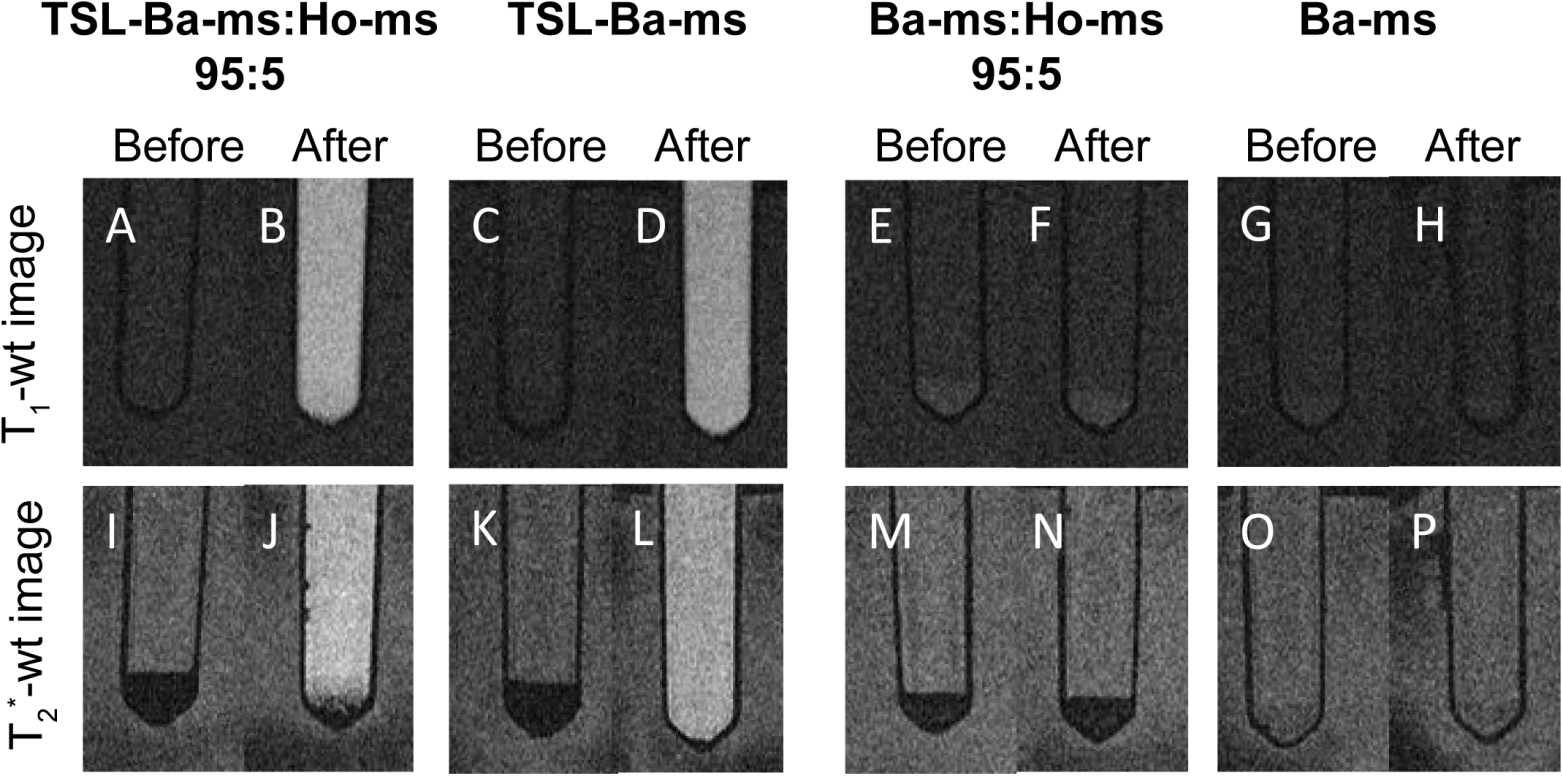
T_1_ and T_2_*-weighted MR images of TSL-Ba-ms or Ba-ms mixed with or without Ho-ms (ratio 95:5) before mild hyperthermia. T_1_ and T_2_*-weighted MR images of TSL-Ba-ms or Ba-ms mixed with or without Ho-ms (ratio 95:5) before mild hyperthermia and after incubation at 42°C for 15 minutes. Signal enhancement in the supernatant after mild hyperthermia indicates release of [Gd(HPDO3A)(H_2_O)] in the T_1_-wt image. The holmium ions appear hypointense in the T_2_*-wt image due to local distortion of the magnetic field.
